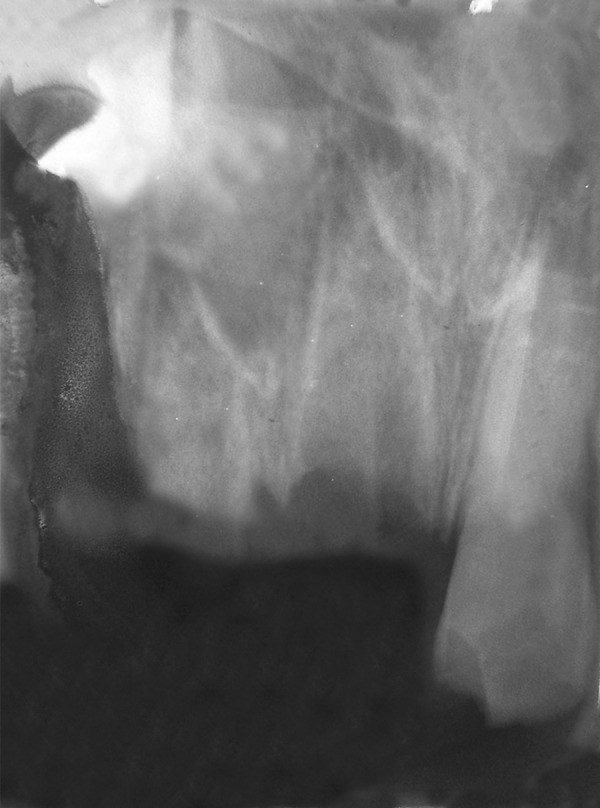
Preoperative intraoral radiograph showing empty alveolar sockets
